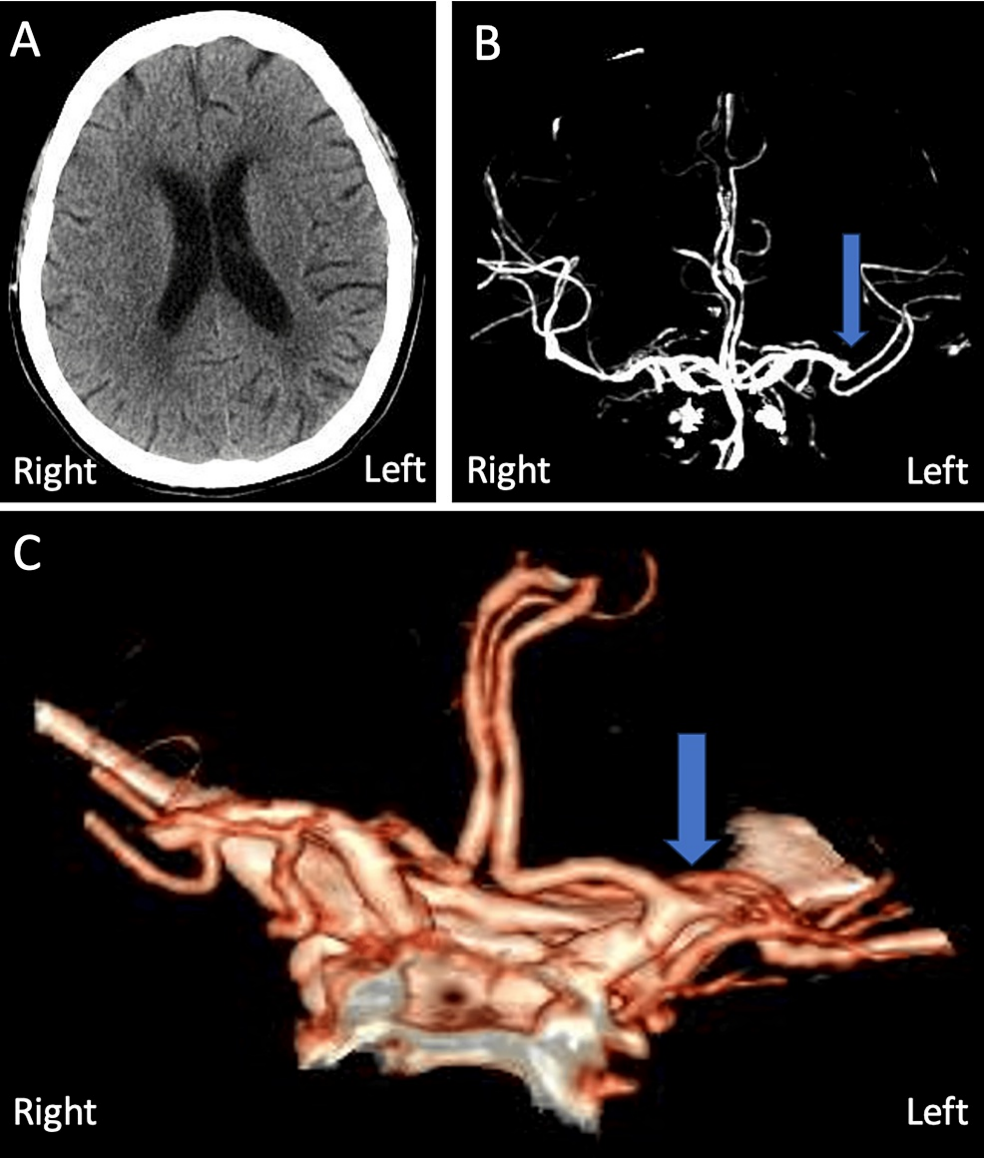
CT images upon initial presentation A) Axial non-contrast image. B) Coronal CT angiography image. C) 3D reconstruction of coronal CT angiography. Blue arrows show the location of the middle cerebral artery occlusion CT: computed tomography
